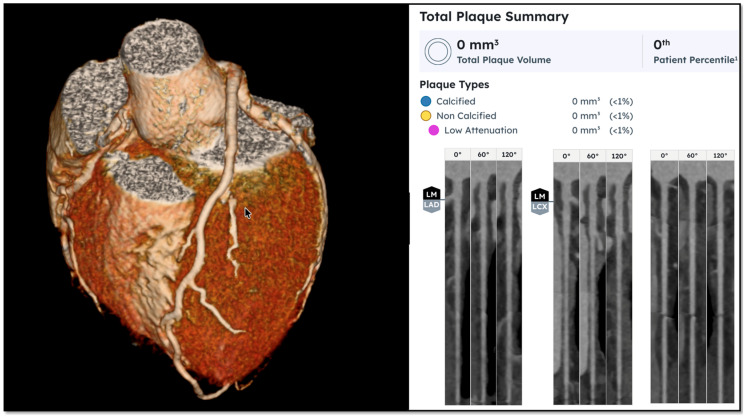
Computed tomography angiography imaging obtained of patient N’s heart, 6 years and 8 months after he commenced a ketogenic diet and developed the lean mass hyper-responder phenotype with total cholesterol over 700 mg/dL (LDL cholesterol 574 mg/dL). Quantification of atherosclerotic plaque by Heartflow^®^ AI identified 0 mm^3^ of plaque volume, placing patient N in the lowest percentile for plaque development.
